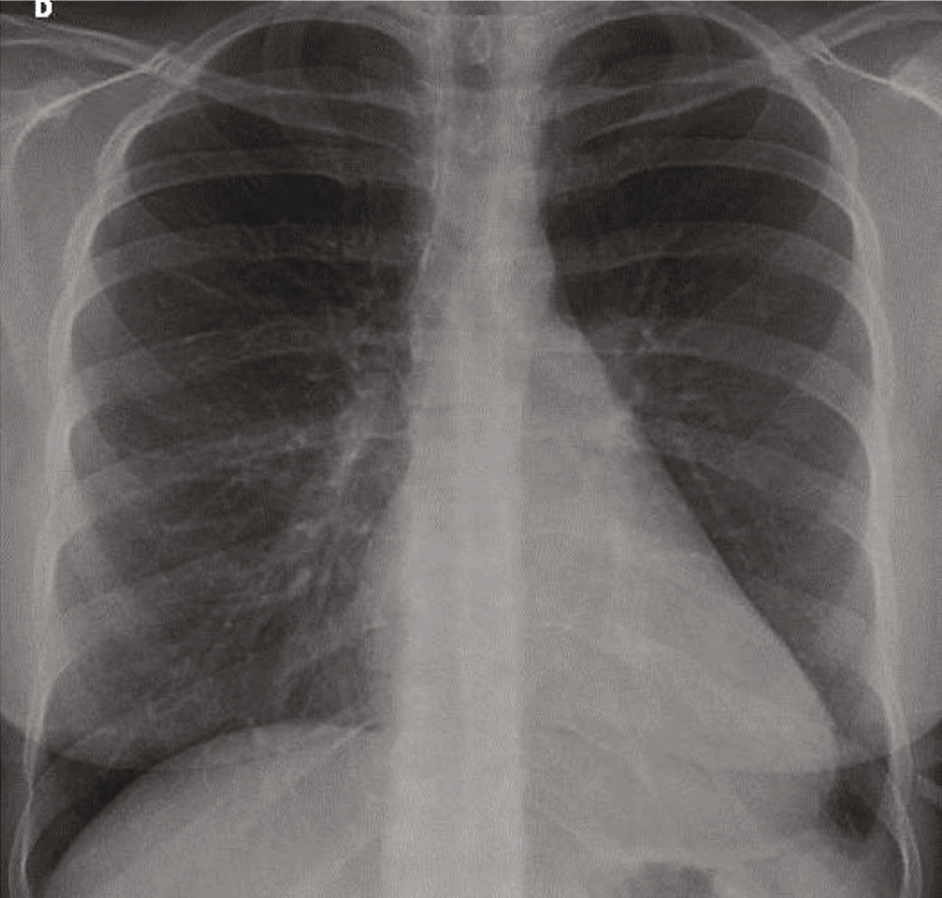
Chest X-ray showing enlargement of cardiac silhouette.
